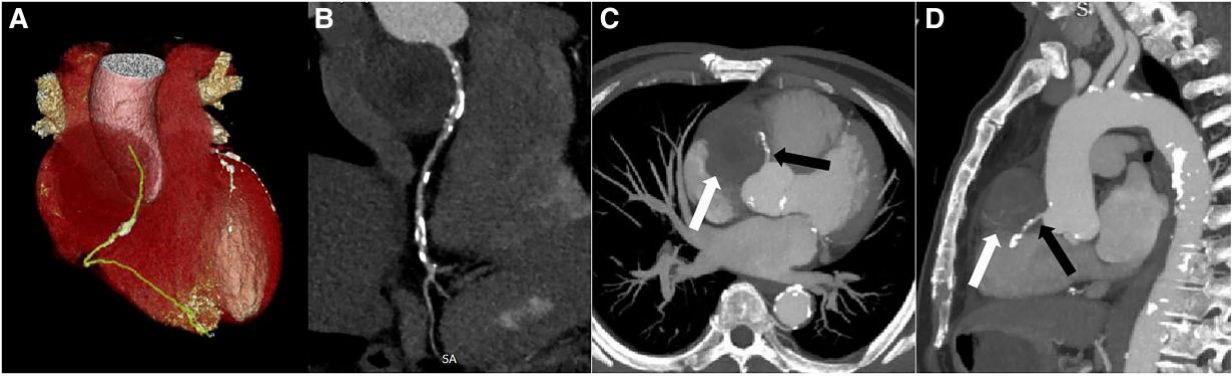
Coronary computed tomography angiography showed the tumour in the right atrioventricular sulcus area, adjacent to the pericardium and the right atrioventricular sulcus. (*A*) Volume rendering images provide a 3D visualization of the tumour located in the right atrioventricular groove. The line represents the right coronary artery. (*B*) Curved planar reformation images indicate that the right coronary artery follows a normal course. (*C*, *D*) Maximal intensity projection images demonstrate that the tumour compresses the proximal segment of the right coronary artery (black arrow: tumour; white arrow: compressed coronary artery).
